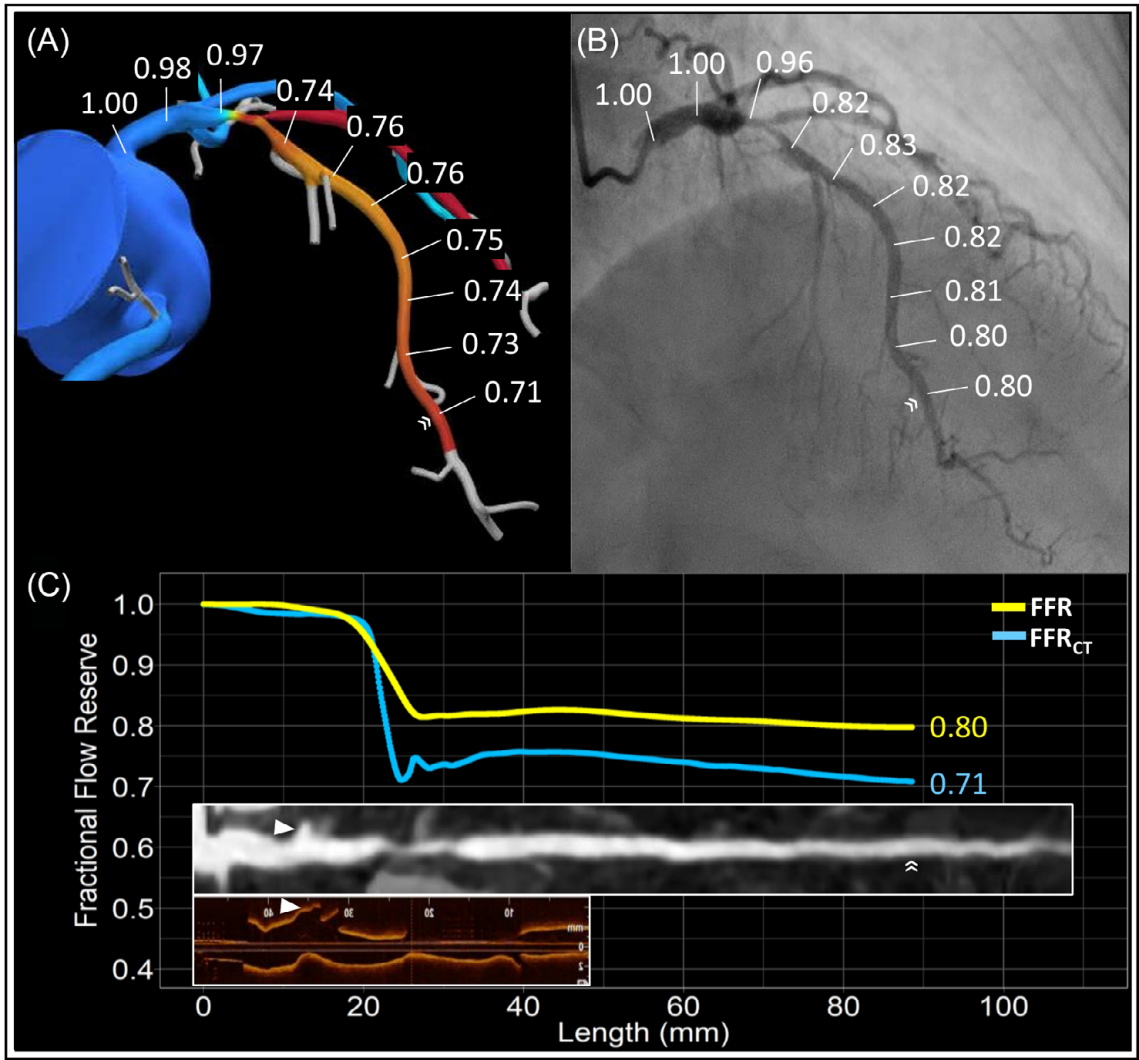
Co‐registration of FFR_CT_, FFR, Coronary CTA and OCT. Panels A and B show a left anterior descending artery with the corresponding FFR_CT_ and FFR. The FFR_CT_ and FFR values along the vessel are used to generate pullback curves shown in panel C in blue and yellow, respectively. The white double arrows point the location of the pressure wire sensor used to co‐register invasive and non‐invasive functional data. On the bottom of panel C, a CT straight multiplanar reconstruction and OCT longitudinal view are co‐registered with the physiologic data. The white arrow heads show the position of side branches used to co‐register OCT and coronary CTA. Coronary CTA coronary computed tomography angiography. FFR_CT_, fractional flow reserve derived from CT. FFR Fractional flow reserve; OCT, optical coherence tomography
